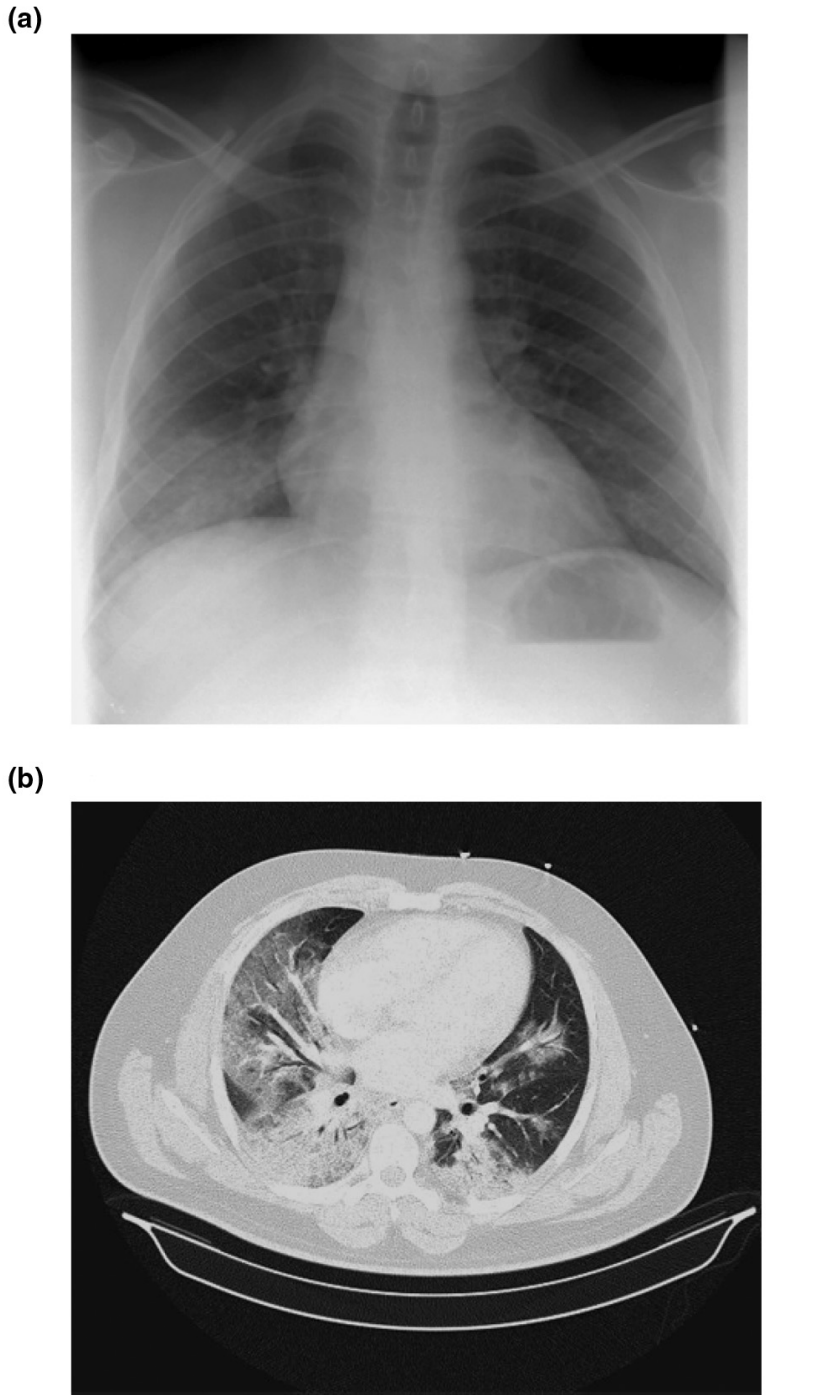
Radiological findings in patients with a viral (H1N1)v pneumonitis. (a) Radiograph and (b) computed tomography scan of the lung in a patient with viral pneumonitis.
